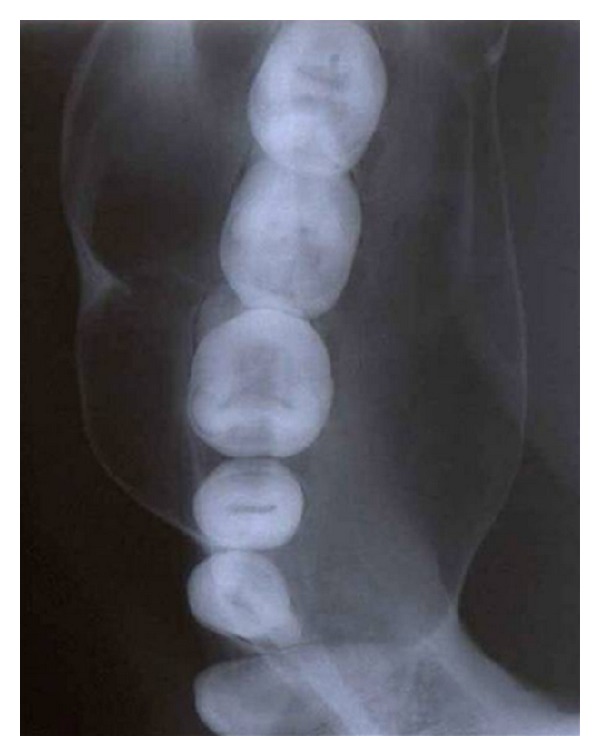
Lateral occlusal radiograph of right side mandible showing expansion of buccal and lingual cortices.
